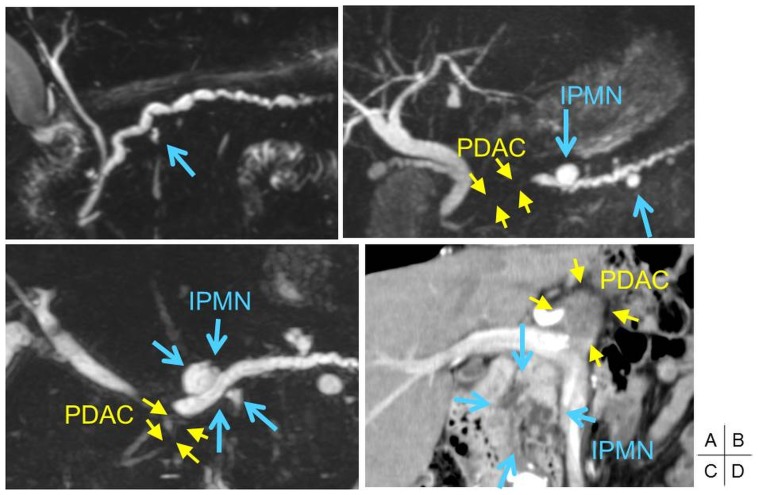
Radiological imaging of pancreatic ductal adenocarcinoma (PDAC) concomitant with intraductal papillary mucinous neoplasm (IPMN). A. A case of CP with a small cyst (blue arrow) that had the *GNAS* mutation (Fig. 1B) in pure pancreatic juice on magnetic resonance cholangiopancreatography (MRCP) imaging. B–D. PDAC with *GNAS* mutations distinct from concomitant IPMN. In cases 30 (B) and 31 (C), MRCP imaging revealed stenosis in the MPD in the pancreatic head near the PDAC (yellow arrowhead). The IPMN was located in the pancreatic body (blue arrow). Computed tomographic imaging in case 28 (D) revealed that the PDAC was located in the pancreatic body (yellow arrowhead), whereas the IPMN was located in the pancreatic head (blue arrow). A list of cases B–D is shown in Table 4.
